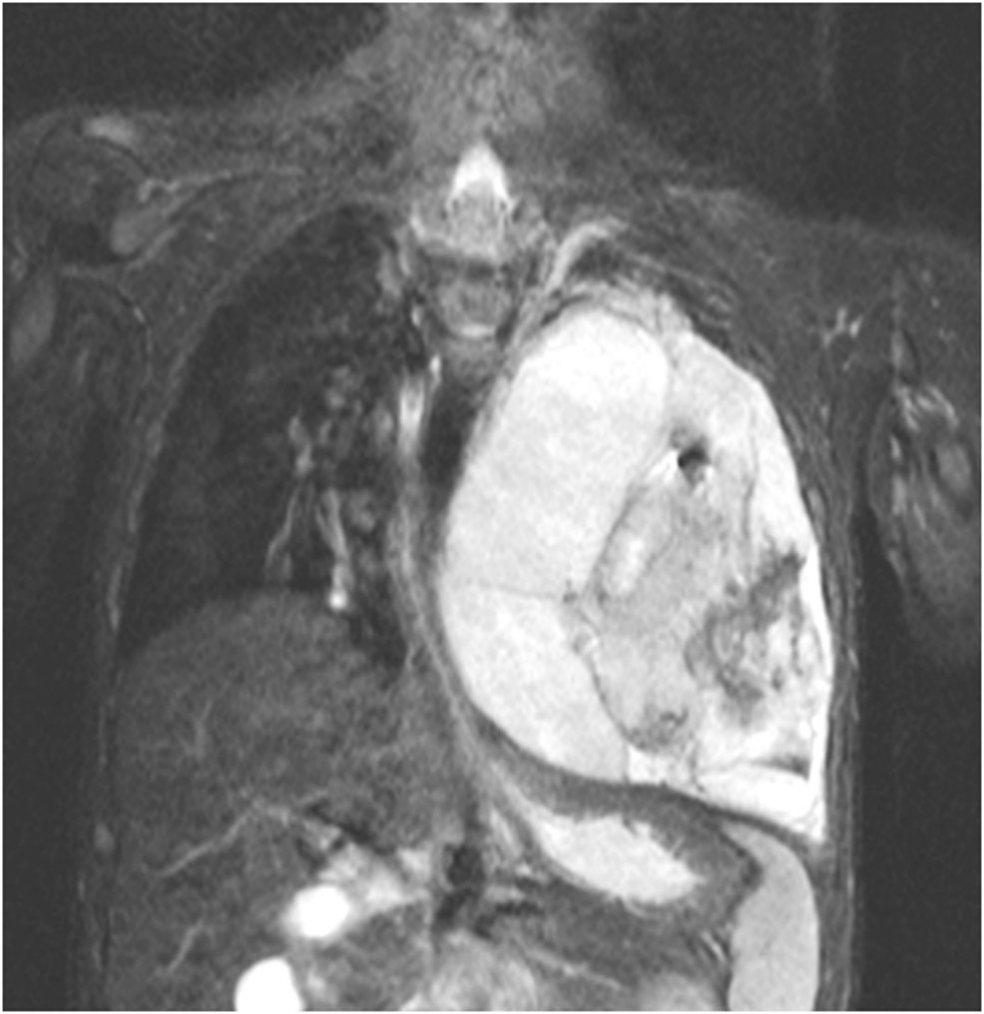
Lung MR in a 2.5-year-old boy with pleuropulmonary blastoma in DICER-1 syndrome. Coronal fat-suppressed T2-weighted turbo spin-echo image (MR parameters in Table 2) shows a partially cystic, partially solid tumor filling the entire left hemithorax
